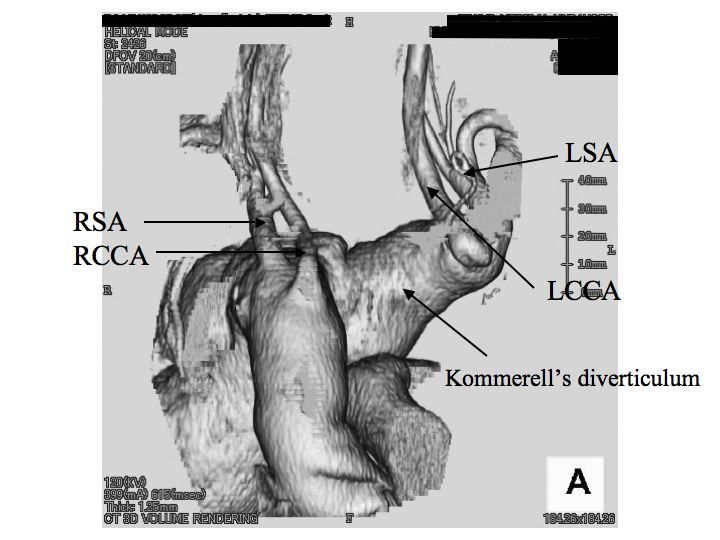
3D CT scan showing the Kommerell's diveticulum and the anomalous pattern of the arch branches.
